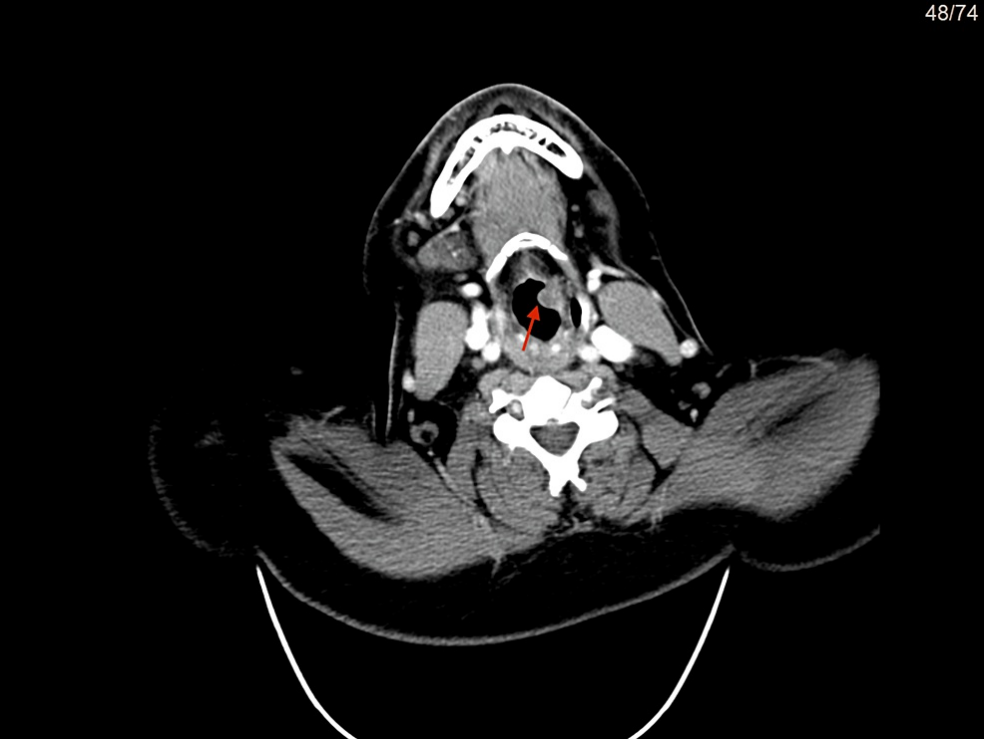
CT scan in axial view The red arrow shows thickening with a small polyp bulging at the left aryepiglottic fold with enlargement of the ipsilateral pyriform sinus.
